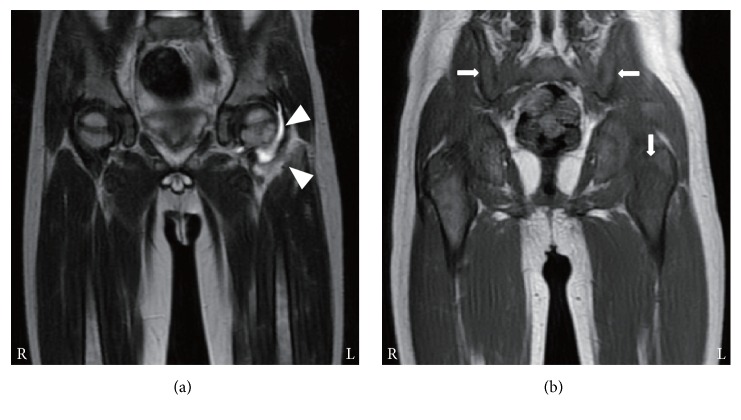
MRI findings in case 1. At 12 days after the disease onset, (a) T2-weighted image shows accumulation of synovial fluid and high signal intensity around the left hip joint (arrowheads) and (b) T1-weighted image depicts low signal intensity areas of the bone marrow in the ilia and the epiphysis of the left femur (arrows).
